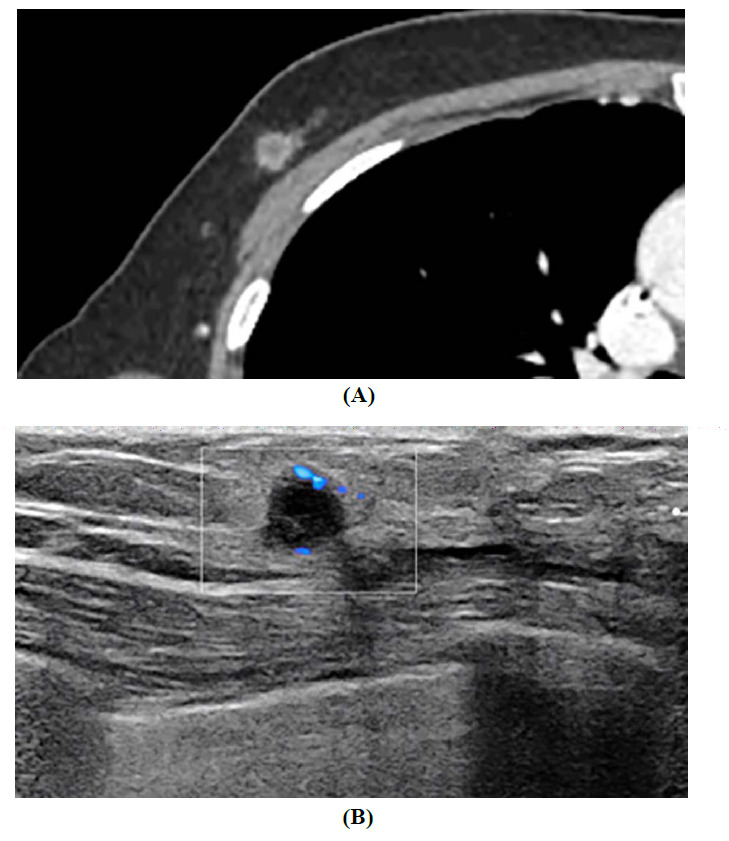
Findings of imaging study of the patient with synchronous primary pure squamous cell carcinoma and invasive ductal carcinoma of the breast. (A) Five months post-operation for IDC of the breast. Chest computed tomography showed a 0.9 x 0.9 x 0.7 cm sized rim enhancing mass with a round shape and relatively circumscribed margin near the previous operation site of the right breast. (B) Breast ultrasound identified a 0.7 x 0.5 x 0.8 cm complex cystic and solid mass with an echogenic rind at the same site, along with increased vascularity on Doppler sonography.
